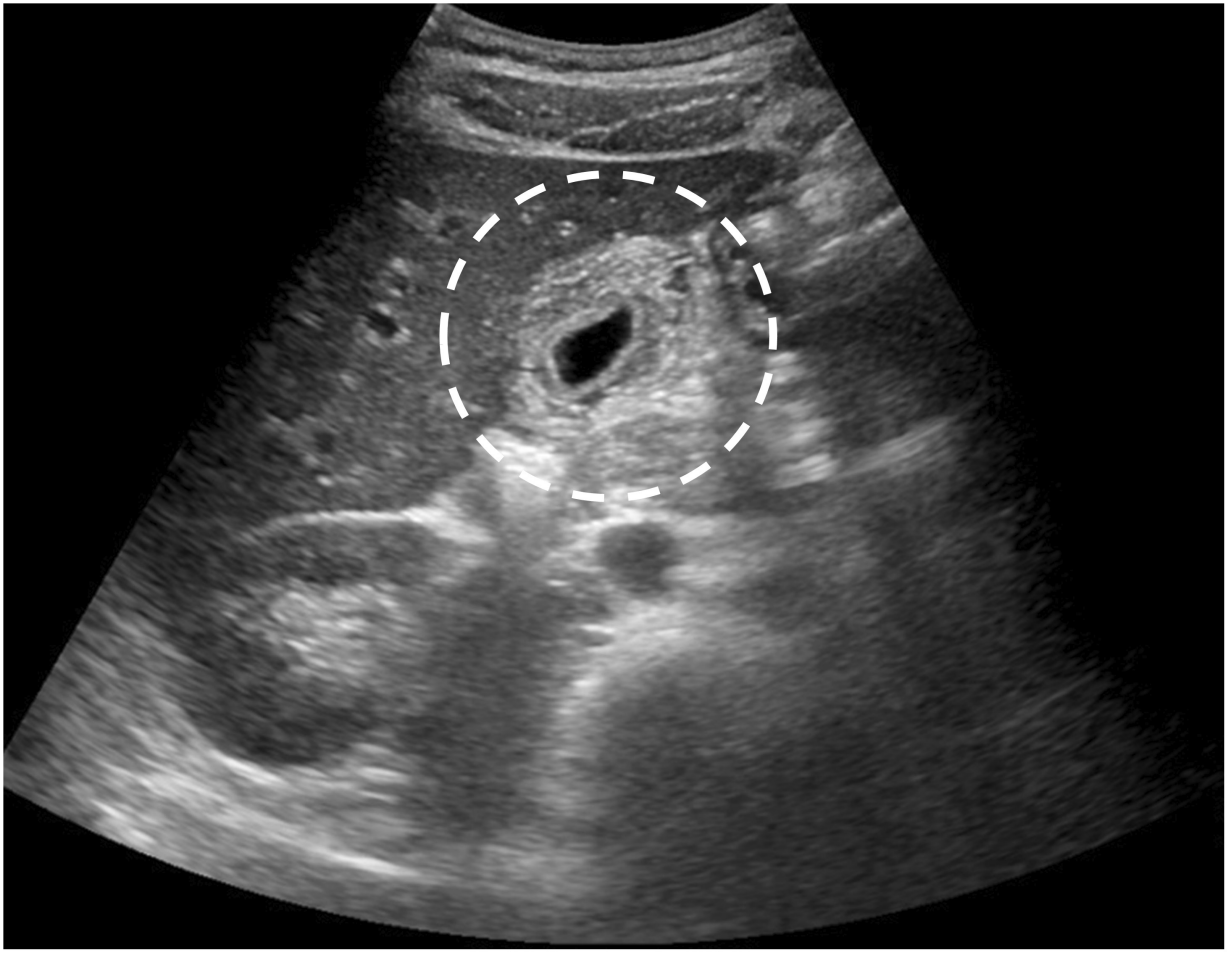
Abdominal ultrasonography. The gallbladder wall is fully thickened around the circumference (dashed circle).
